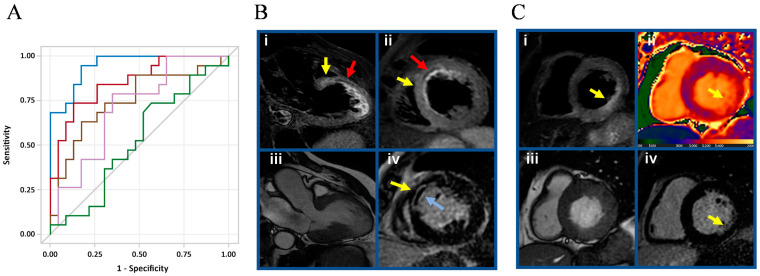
CD41–CD61 expression and size discriminated patients with MVO/MSI. (A) ROC analysis was used to evaluate the ability of sEV profile (concentration, dimension, and CD41–CD61 expression) to identify patients with MVO and/or low MSI. Violet line: CD41–CD61 expression; brown line: dimension; green line: concentration; red line: troponin peak; blue line: Model including all the variables of sEV profile and troponin. (B) Patient with unfavorable CMR and sEV profile. A 69-year-old man with acute anterior STEMI: Three-chamber long axis view (i) and mid ventricle short axis view (ii) T2-weighted triple inversion recovery (TIR T2) images showing myocardial edema (yellow arrow) and myocardial hemorrhage (red arrow) at anteroseptal wall. (iii) Three-chamber long axis view cine image at end-systole, showing akinesia at mid-to-apical anteroseptal wall and apical inferolateral wall. (iv) Mid ventricle short axis view late gadolinium enhancement (LGE) image showing transmural enhancement (yellow arrow) and microvascular obstruction (light blue arrow) at anteroseptal wall. (C) Patient with favorable CMR and sEV profile. A 50-year-old man with acute inferolateral STEMI. (i) Basal ventricle short axis view TIR T2 image showing myocardial edema (yellow arrow) at inferolateral wall. (ii) Basal ventricle short axis view native T1 mapping image showing focal increased T1 values (up to 1372 msec; values at remote myocardium 1004 ± 30 msec) in line with focal myocardial damage (yellow arrow) at inferolateral wall. (iii) Basal ventricle short axis view cine image at end-systole, showing mild hypokinesia at inferolateral wall. (iv) Basal ventricle short axis view LGE image showing subendocardial enhancement involving 25–50% wall thickness (yellow arrow).
